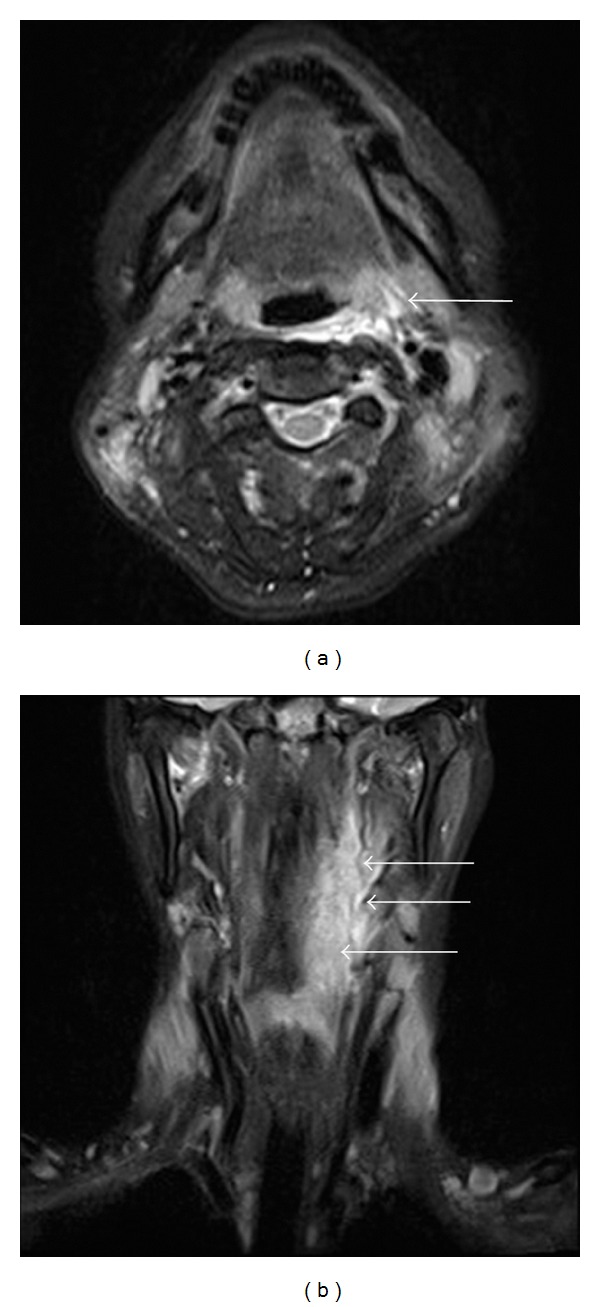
MRI of the neck: (a) T2WI axial section and (b) T2WI coronal section T2 hyperintensities are observed in the bilateral neck muscles, predominantly in the left pharyngeal constrictor muscles (arrows).
